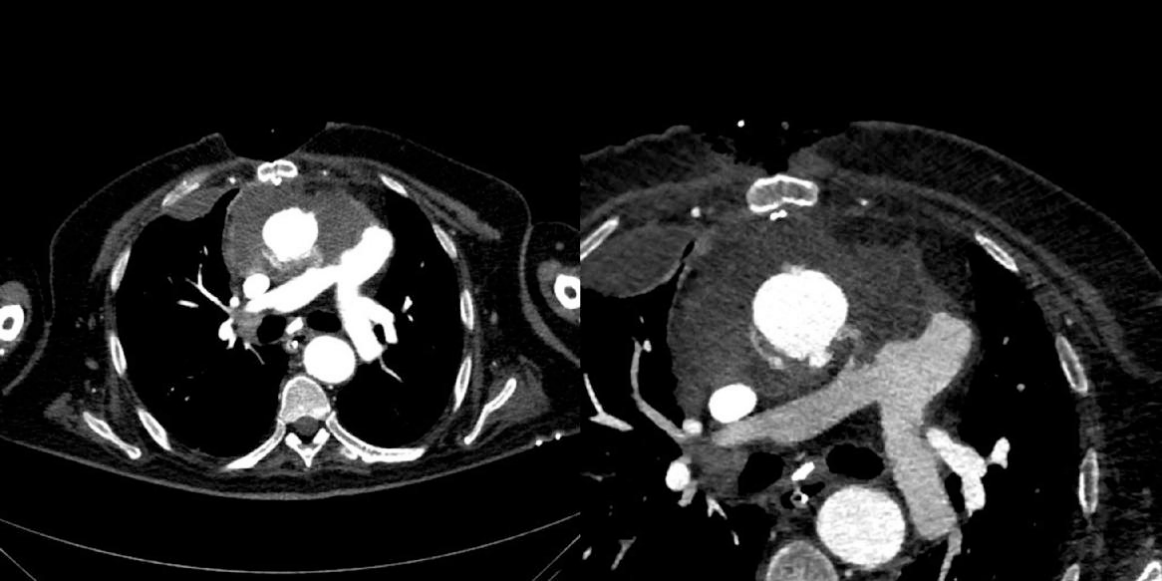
Post-operative contrast computed tomography angiography images in oblique axial reconstruction demonstrating the complete resolution of the aortopulmonary fistula (APF).
